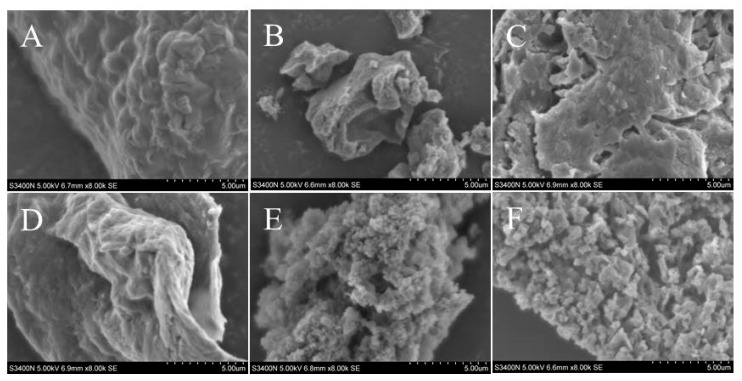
The polysaccharides morphology using different extraction methods with 8.00k SEM. (A) ultrasound-assisted DES-6 extraction; (B) decoction piece; (C) ultrasonic water extraction; (D) distilled water extraction; (E) hot water reflux; (F) thermal extraction.
